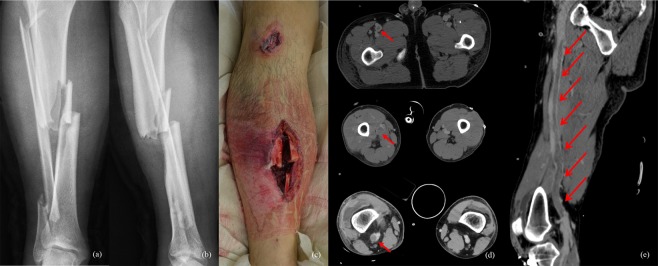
A 41-year-old male patient with right tibio-fibular open fracture and left ankle fracture after a motorcycle accident developed right lower-extremity severe swelling and pain during passive ankle and toe motion (a–c). CT angiography was performed. A massive thrombus in the right common femoral vein and calf were confirmed (red arrow) and chemical treatment was administered continuously (d,e).
